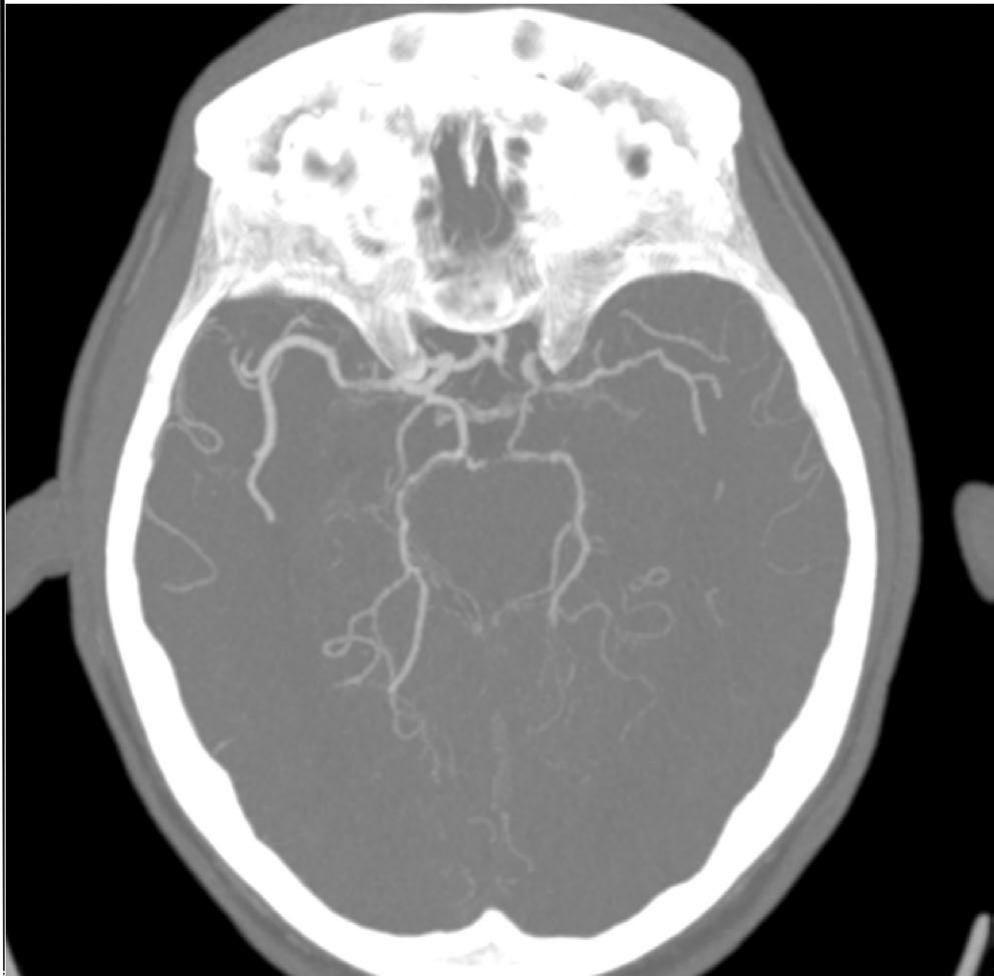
FIGURE 2 CT angiography showed multifocal intracranial arterial narrowing and beading, involving both the anterior and posterior circulation.
